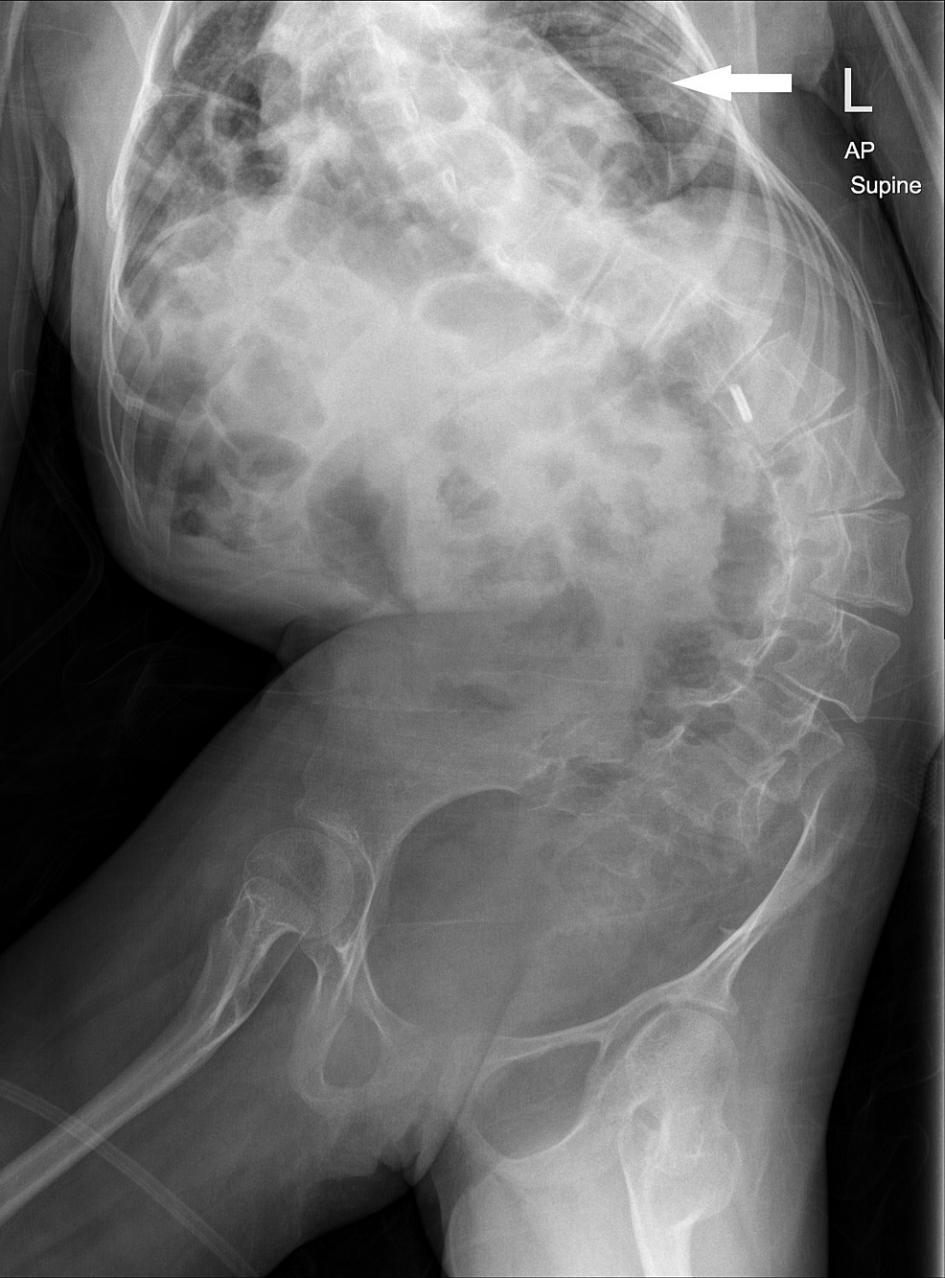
Chest and abdominal X-ray showing thoracolumbar scoliosis and left-sided diaphragmatic hernia with a hypoplastic lung (white arrow).
